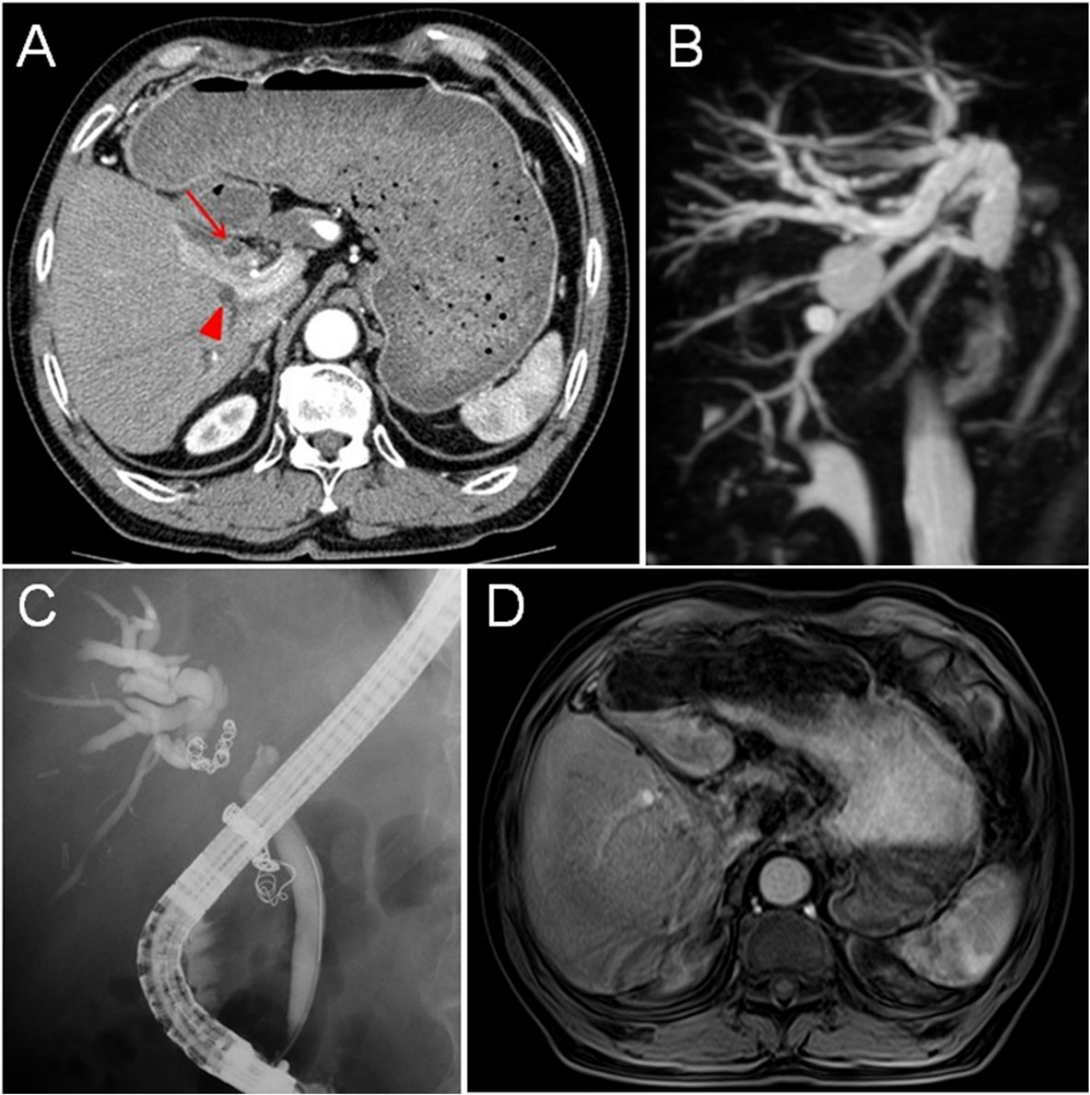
Preoperative imaging findings. a Computed tomography showed that cholangiectasis was observed in both anterior (*arrow*) and posterior (*triangle*) segments of the right hepatic lobe, but no definitive mass lesions were found in the liver. b Magnetic resonance cholangiopancreatography showed cholangiectasis from the intrahepatic bile ducts to the middle segment of the common bile duct. c Endoscopic retrograde cholangiopancreatography showed that the common bile duct was obstructed by a tumorous lesion covering the upper and middle segment of the common bile duct. d Dynamic liver magnetic resonance imaging showed that the tumor became ambiguous due to the influence of the biliary drainage, and it had poor contrast effect in all contrast phases (the displayed image is early phase image). No definitive mass lesions except for simple cysts were found in the liver
